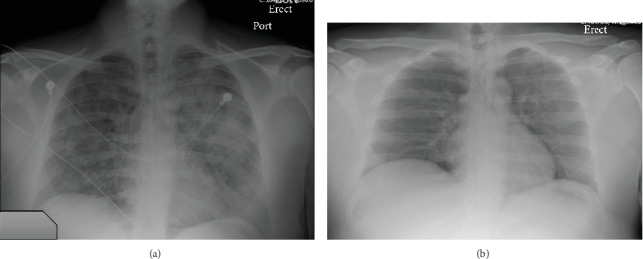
(a) Single-view anterior-posterior chest X-ray on day #1 showed diffuse bilateral lung opacities. (b) Repeat single-view anterior-posterior chest X-ray on day #6 showed decreased airspace opacities.
